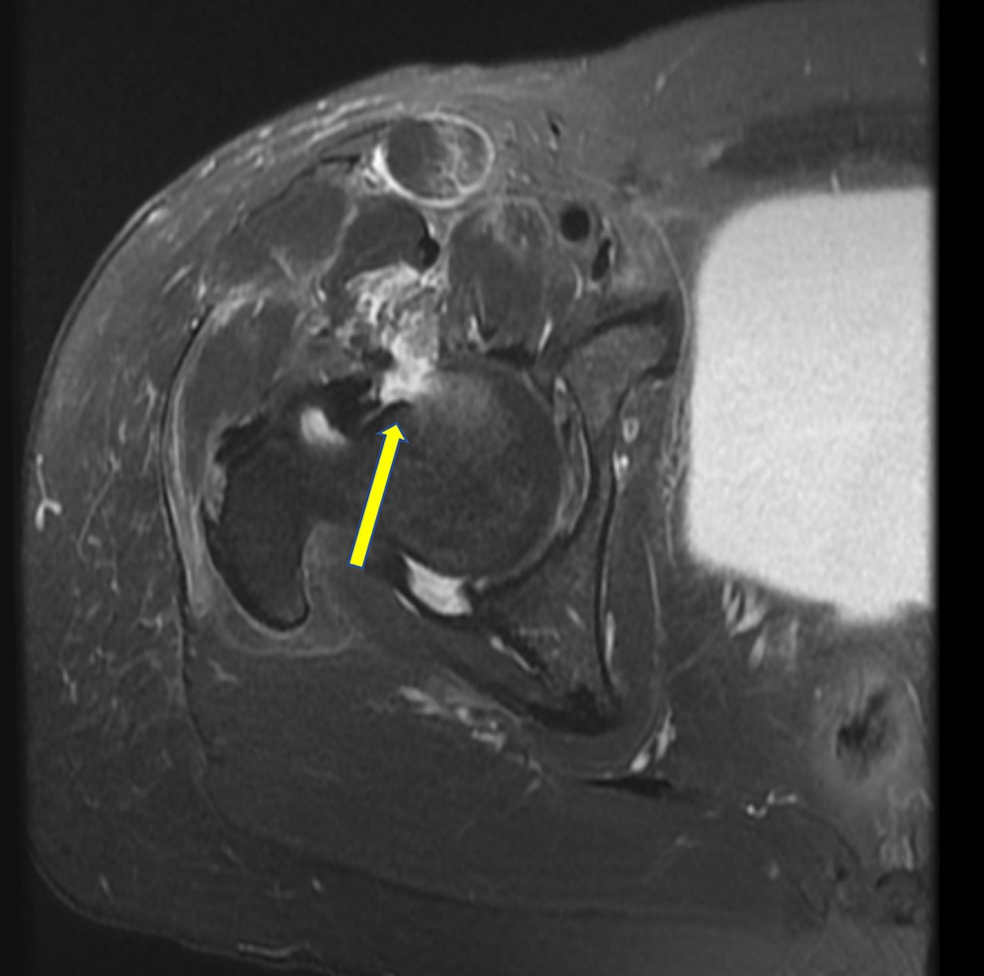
MRI of the right hip. T2-axial fat-suppressed view demonstrates edema-like signal in the anterior femoral head and neck junction suggestive of impaction fracture (yellow arrow).
